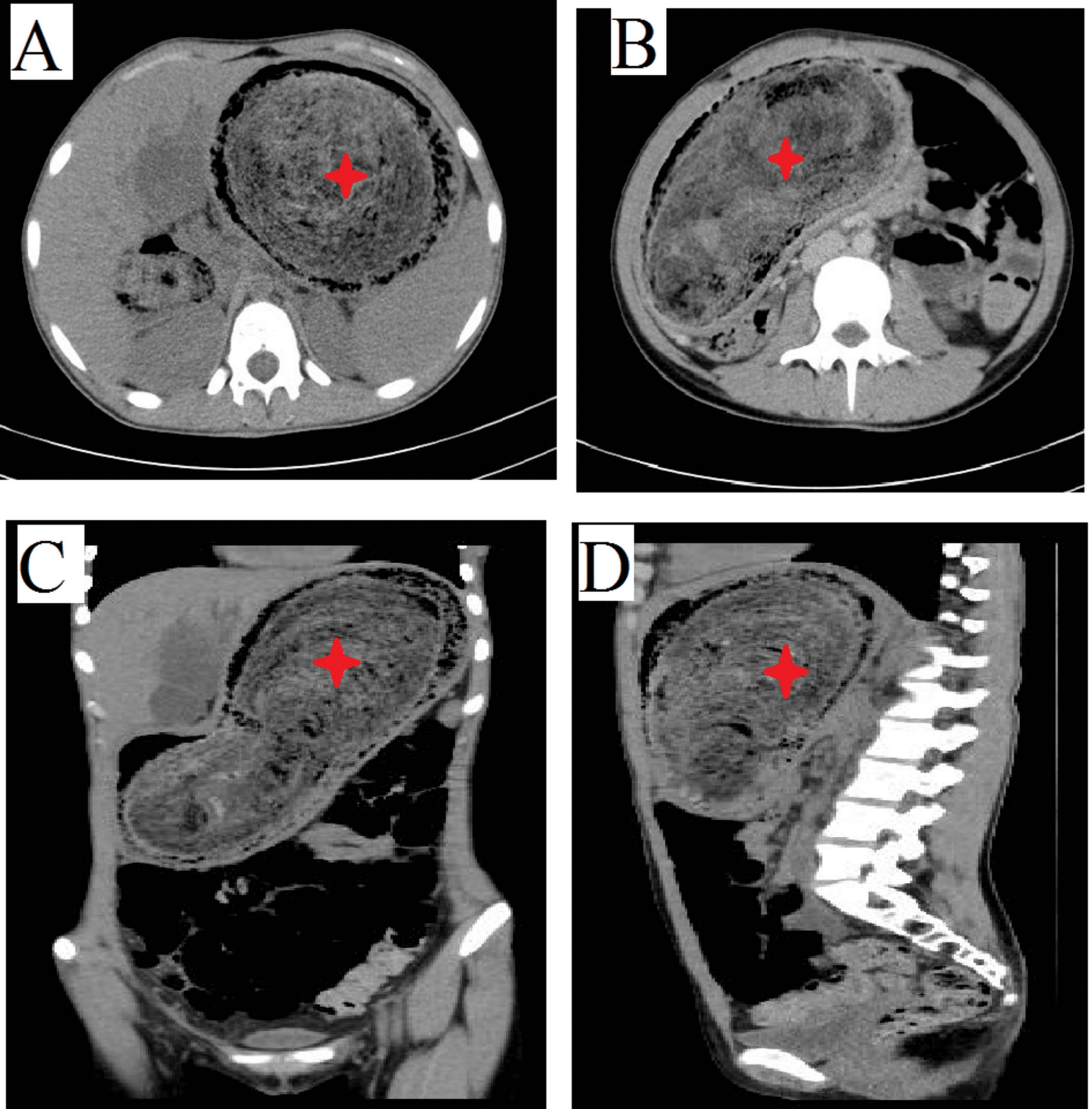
The abdominal computed tomography. It shows the stomach is distended and filled with heterogeneous mass in different views - axial (A, B), coronal (C), and sagittal (D).
